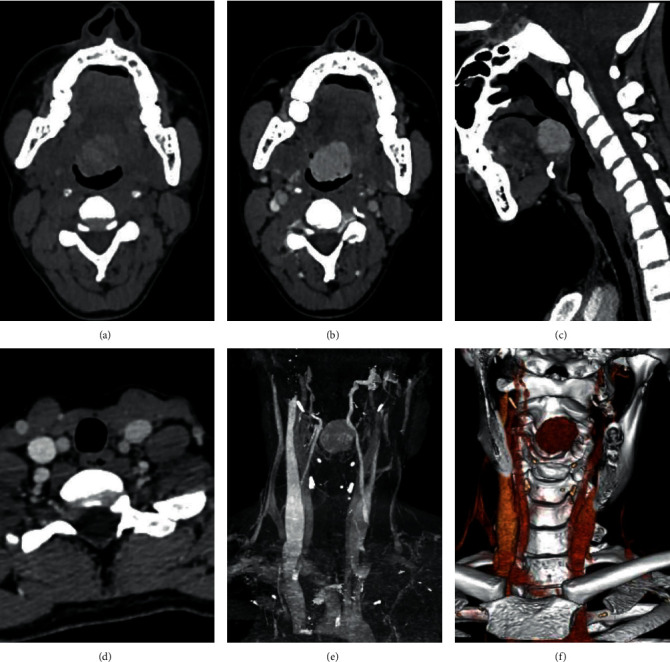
Computed tomography (CT) scan of the neck. Axial noncontrast CT image with a well-defined relatively hyperdense nodule in the midline at the base of the tongue (a), axial contrast-enhanced CT showing homogeneous enhancement (b), sagittal contrast-enhanced CT image with an enhancing nodule at the level of C2-C3 vertebra causing narrowing of the oropharyngeal lumen (c), axial contrast-enhanced CT image below the cricoid cartilage without orthotopic thyroid (d), coronal maximum intensity projection (MIP) image with a nodule in the midline (e) with absent orthotopic thyroid, and volume rendered image with a nodule in the midline (f).
